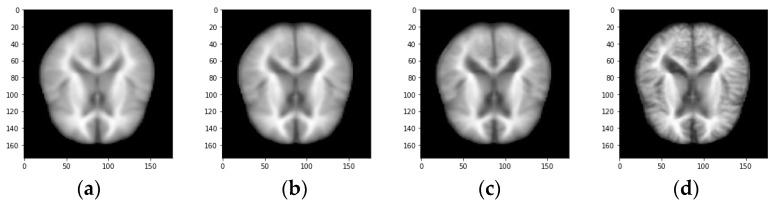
Sample MRI image demented categories. (a) Non-demented image (b) Very mild demented image (c) Mild demented (d) Moderate demented.
